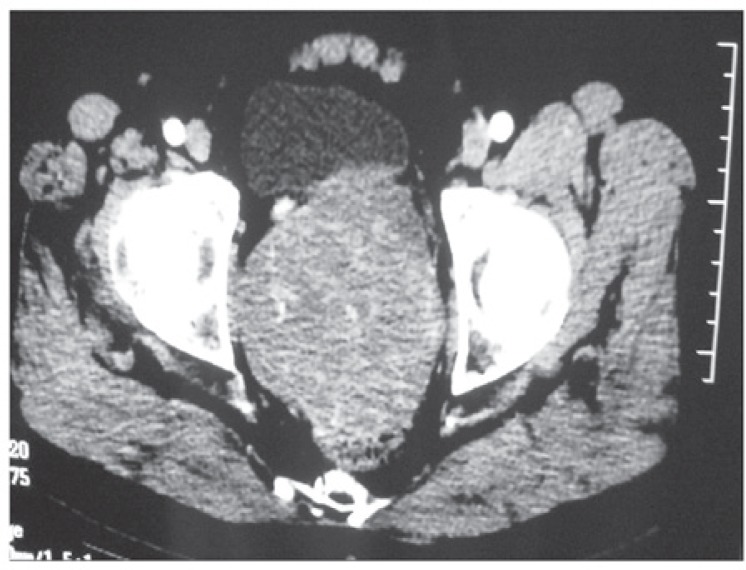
Pelvic computed tomography (CT) scan revealing a heterogenous mass occupying the majority of the prostate.
